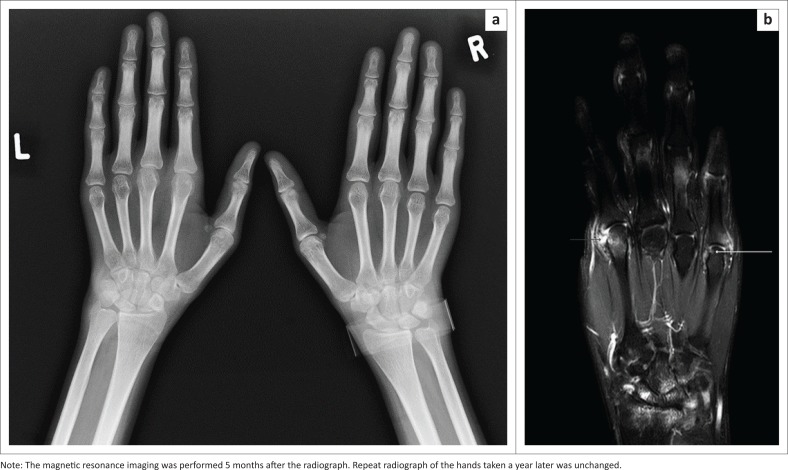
(a) Frontal radiograph of the both hands shows no evidence of erosive disease. (b) Post-contrast fat-suppressed coronal T1-weighted magnetic resonance imaging of the same hand demonstrating an erosion (white arrow) and active synovitis (grey arrow).
